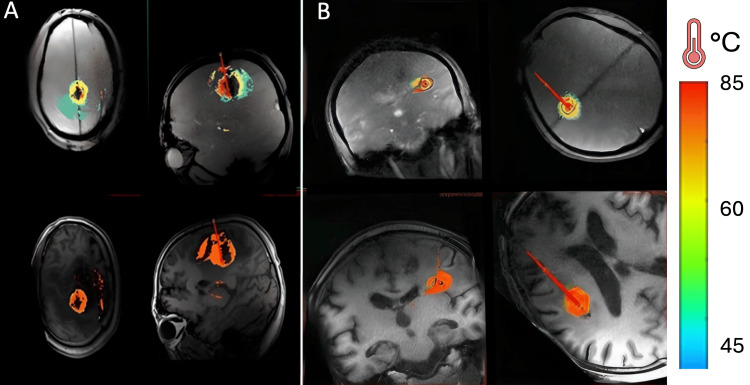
Real-time intraoperative MRI imaging (A) and (B) thermal damage threshold (TDT) of the large and small tumors, respectively, and temperature bar on the left side
